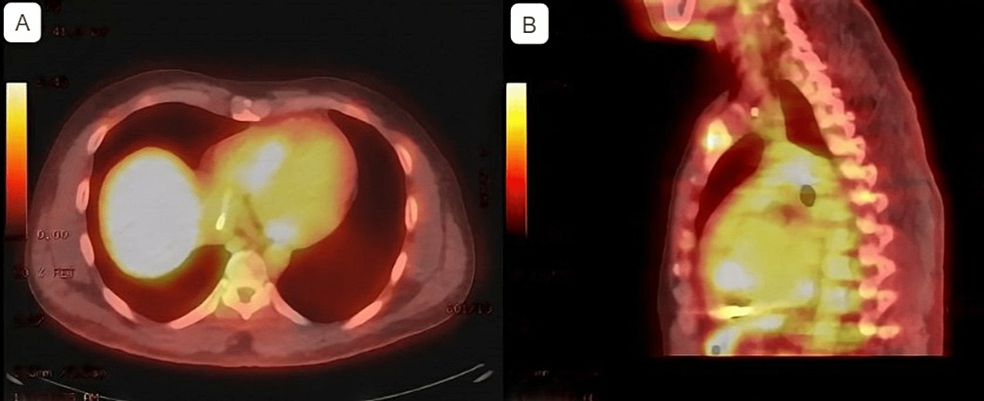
PET/CT scan showing a focal uptake of FDG in the inferior septum, indicating active inflammation A: Transverse section showing increased FDG uptake in the inferior septum B: Sagittal section showing increased FDG uptake in the inferior septum FDG: 18F-fluorodeoxyglucose; PET: positron emission tomography
